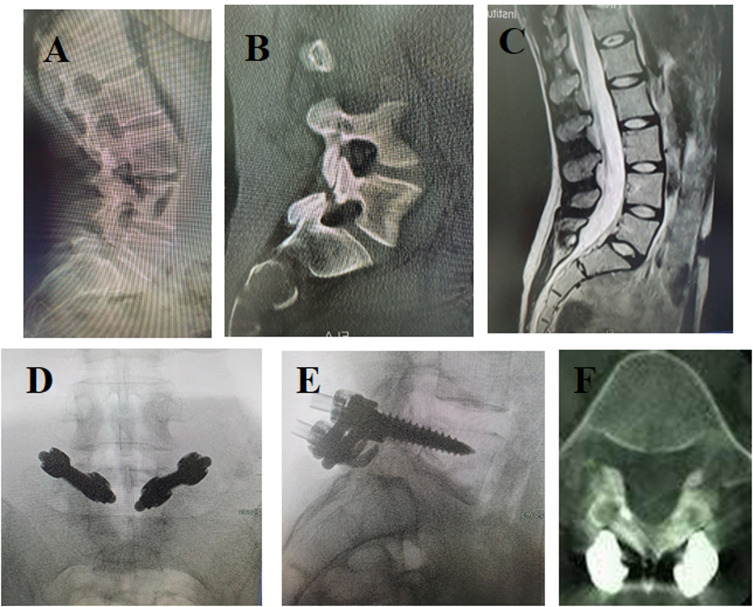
Twenty-six years old female with symptomatic spondylolysis performed pars repair after failed conservative treatment for 6 months. (A) Preoperative plain X-ray, (B) preoperative CT, and (C) preoperative MRI showing spondylolysis at L5. Pedicle screw hook fixation was performed. (D, E) Intraoperative anteroposterior and lateral images. (F) CT at final follow-up showing healing of the defect.
